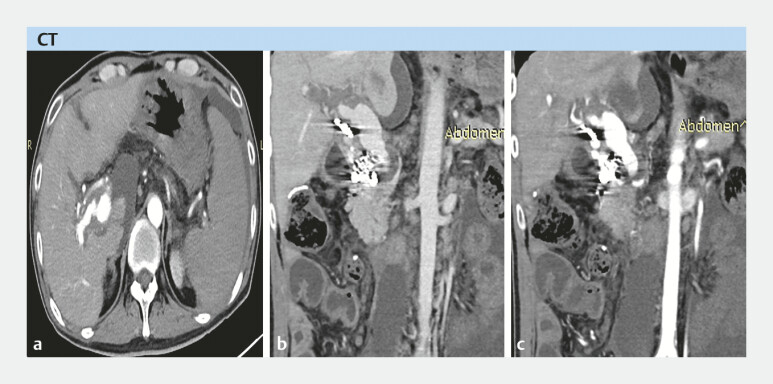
Enhanced computed tomography (CT) showing (
